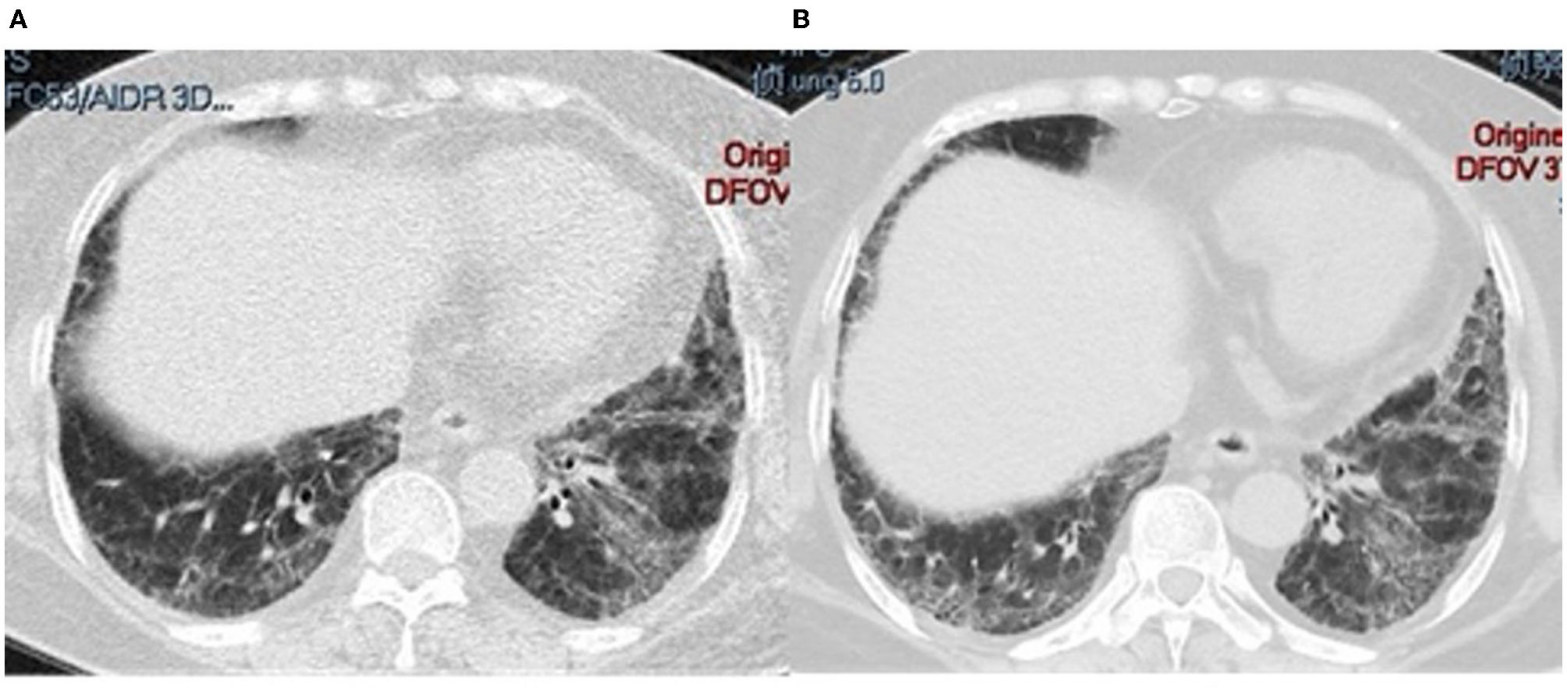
Lung CT changes in a 41-year-old man with PM/Scl-75 and−100. (A) CT at onset; (B) ILD progression after 8 months of treatment with an anti-fibrotic drug.
